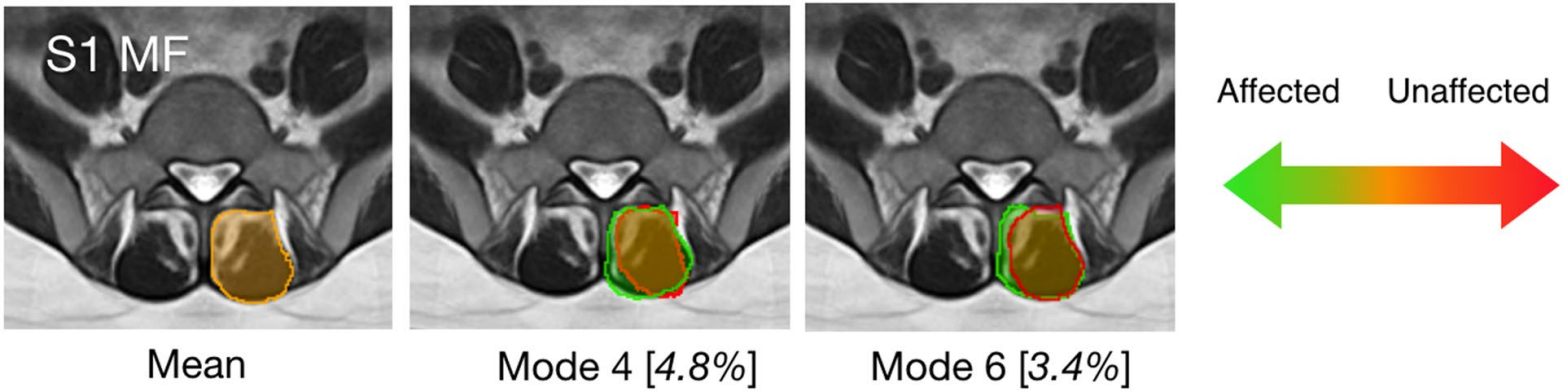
Modes of the multifidus (MF) shape that were associated with the disc herniation at S1. The mean shape is shown in orange overlaid on the population-averaged MRI template. The trend of shape variation towards the muscle being affected is in green and the opposite is in red. For each mode, threefold standard deviations from the mean shape are depicted for both directions of shape variants, and the percentages of shape variance explained are included in [] for all modes.
